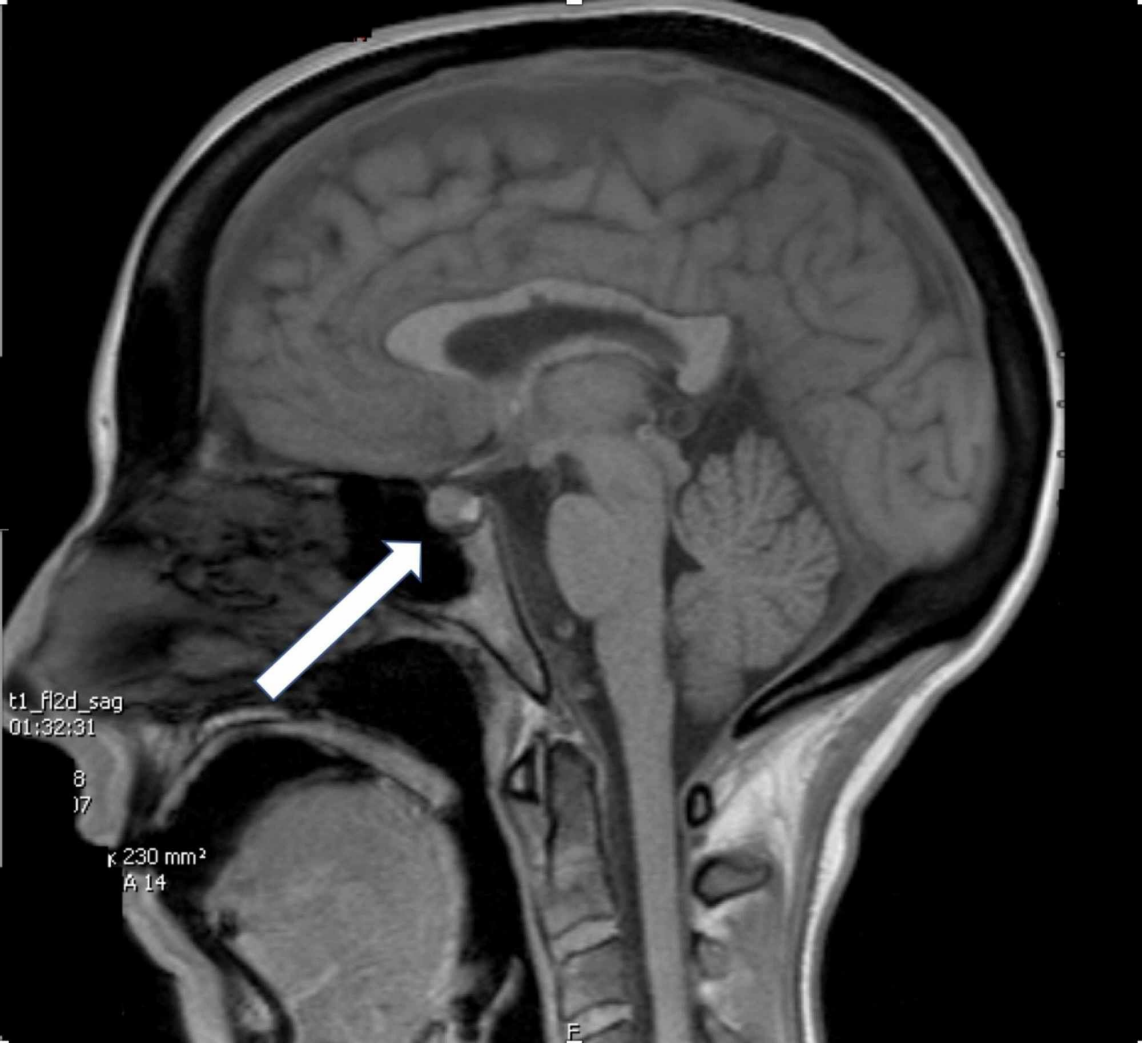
Saggital view of head magnetic resonance imaging (MRI) depicting pituitary bulge (white arrow)
